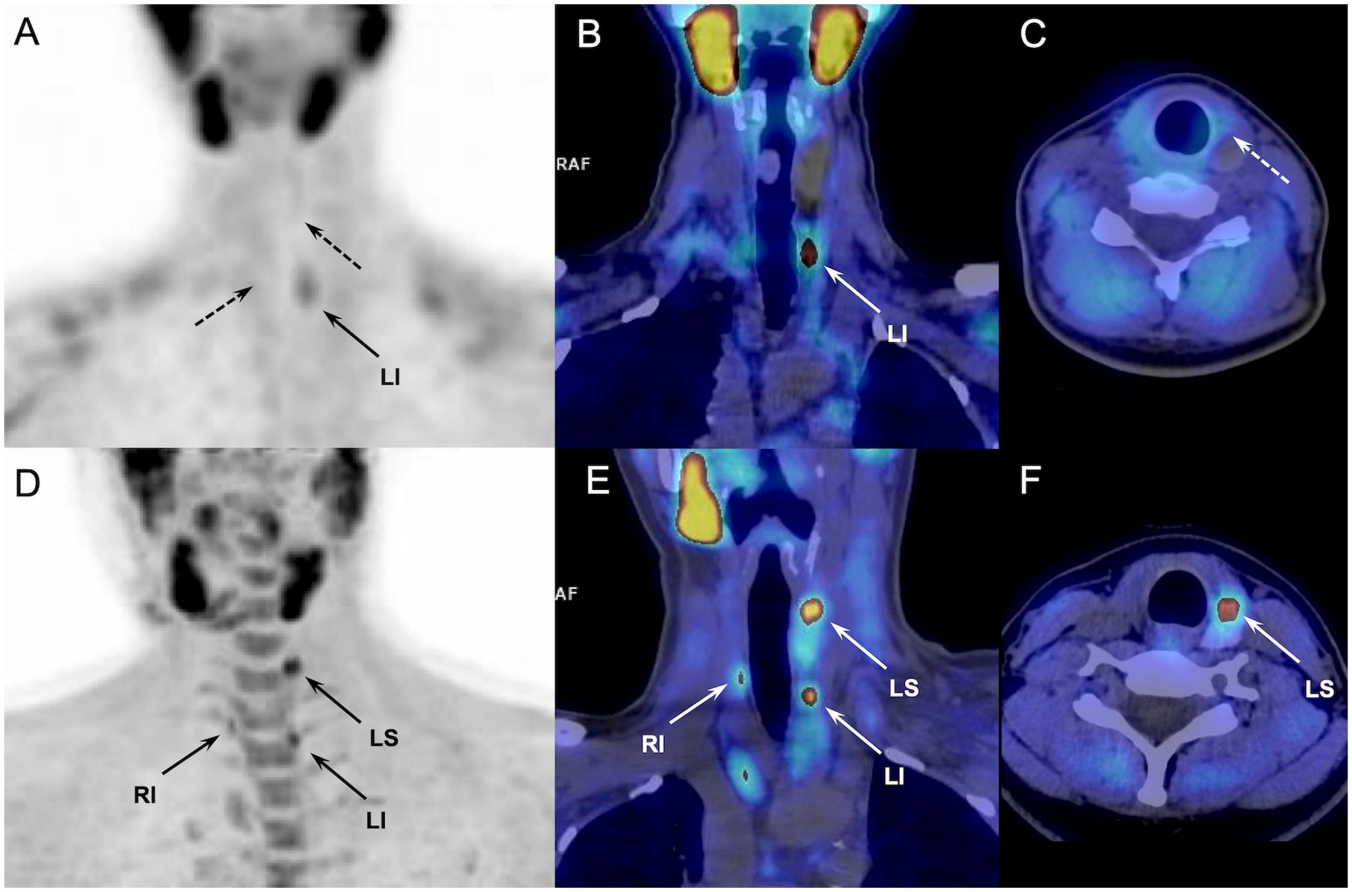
Discordant results of ^99m^Tc-sestamibi parathyroid scintigraphy (upper panel, A-C) and ^18^F-FCH PET/CT (lower panel, D-F) (anterior MIP, coronal and axial fusion images) performed in a 28-y-old MEN1 patient with previous history of superior right parathyroidectomy (patient#7, Table 2). ^99m^Tc-sestamibi scan showed a pathological left inferior parathyroid (arrow). ^18^F-FCH PET/CT confirmed scintigraphy findings and detected 2 more hyperfunctioning glands (arrows, inferior right and superior left) not revealed by scintigraphy, and afterwards confirmed by pathology after surgical excision.
